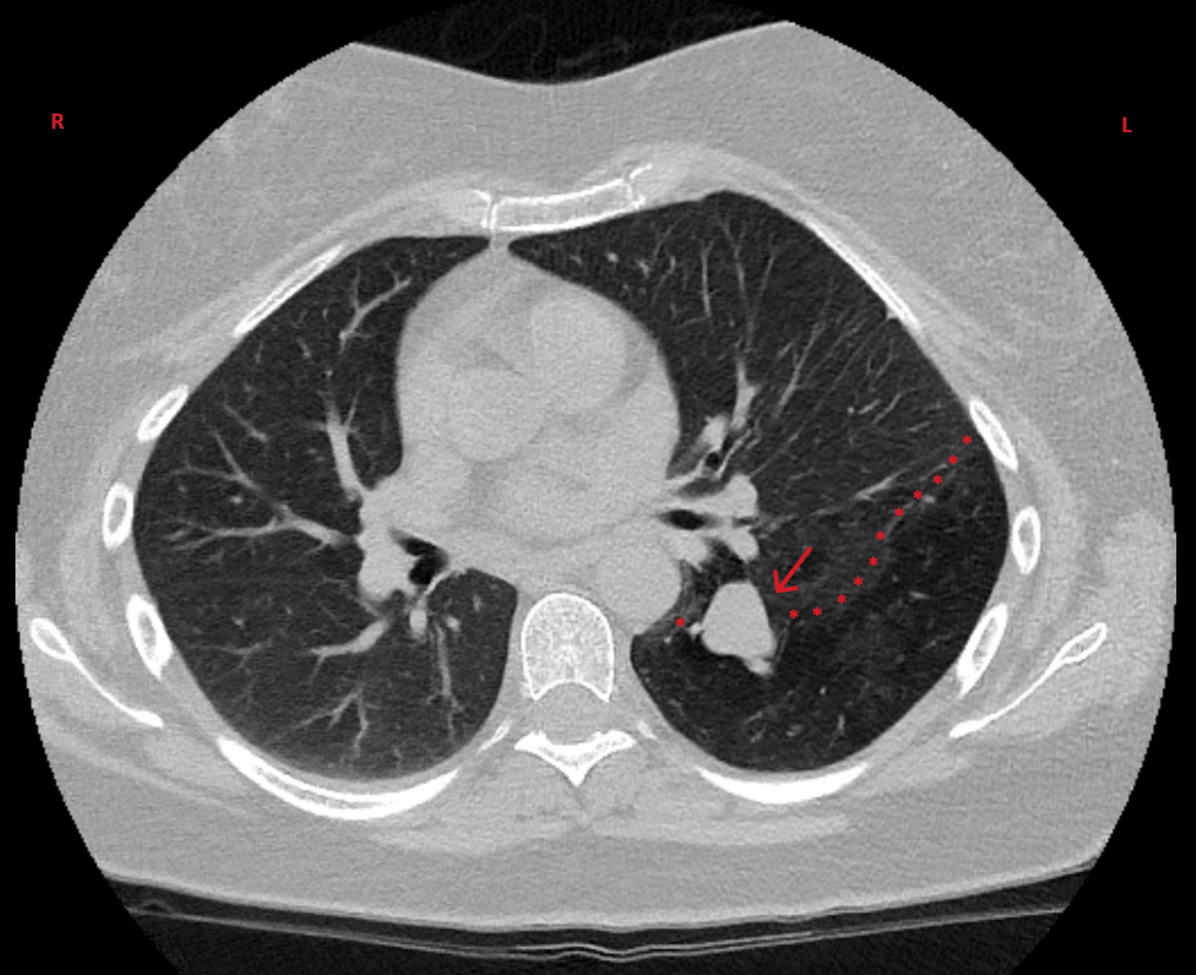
Follow-up computed tomography of the chest three weeks after clinical resolution, revealing a finger-like mucus-filled structure extending from the left hilum (red arrow), measuring up to 13 mm in maximum diameter, without communication to the remaining bronchial tree. Adjacent parenchyma demonstrates marked emphysematous changes (red stars). These findings are characteristic of congenital bronchial atresia. Note the complete resolution of previous consolidation, confirming the inflammatory/infectious nature of the initial findings.
